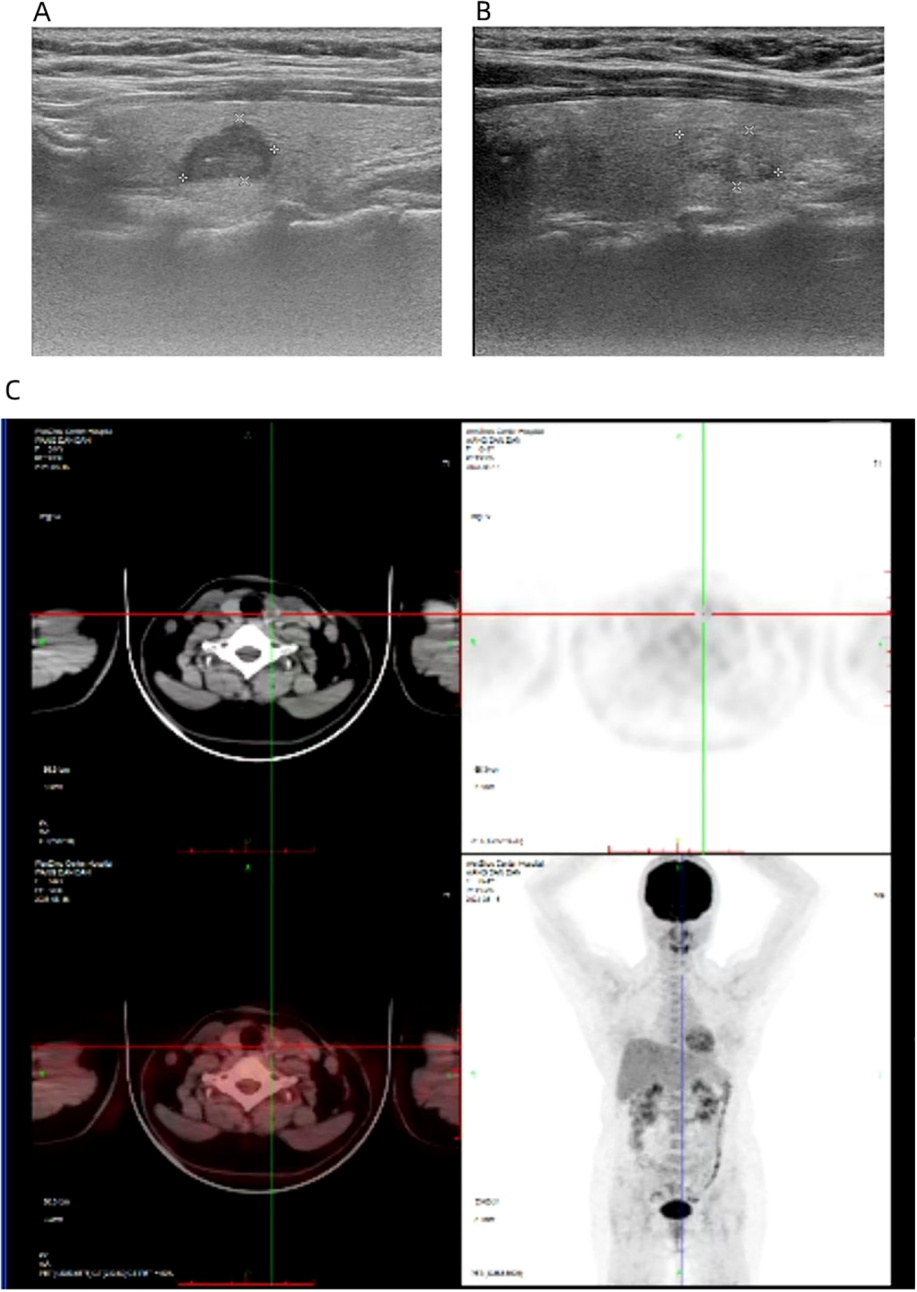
The ultrasound image shows a nodule of 1.2 * 0.8 * 0.9cm in the left thyroid gland (A) and a nodule of 1.3 * 0.7 * 0.9 cm in the right thyroid gland (B). PET-CT revealed an increase in FDG metabolism in the left thyroid nodule, while whole-body imaging showed no abnormal increase in FDG metabolism (C).
